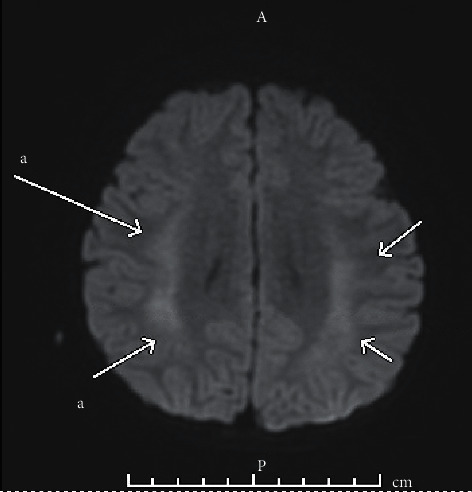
MRI findings on day 2 of hospitalization. Fluid-attenuated inversion recovery axial view shows bilateral frontoparietal lobe diffusion restriction, seen in acute encephalopathy or postictal changes.
